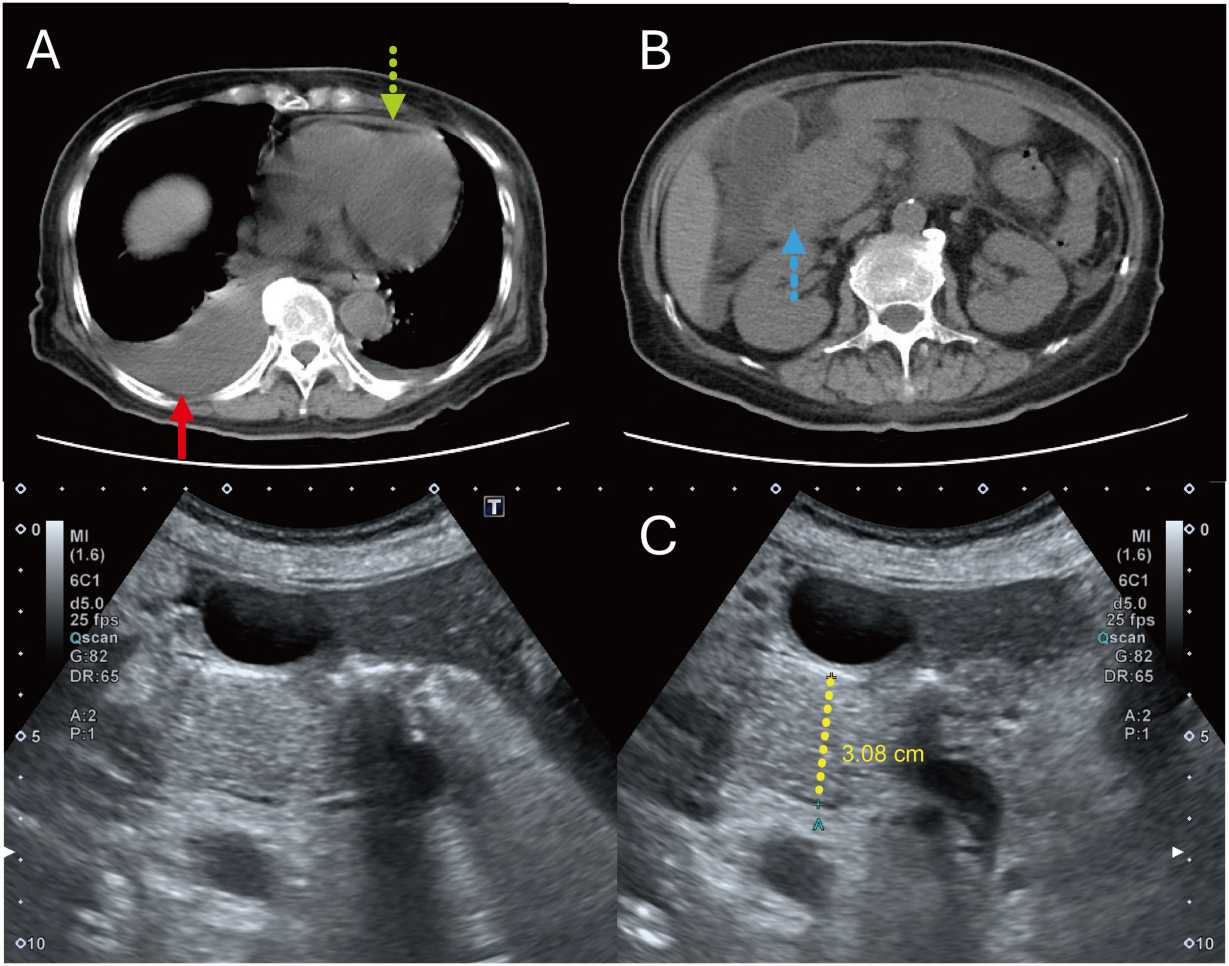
Patient 1, non-contrast CT (PSO 7) and transabdominal ultrasonography (PSO 13). (A) Non-contrast abdominal CT on PSO 7 revealed a pericardial effusion (green dotted arrow) and left and minimal right pleural effusions (red arrow). (B) A 3.3-cm iso-dense lesion at the pancreatic head with surrounding inflammatory edema (blue dashed arrow). (C) Follow-up transabdominal ultrasonography on PSO 13 demonstrated a 3.08-cm isoechoic lesion at the pancreatic head (yellow dotted line).
